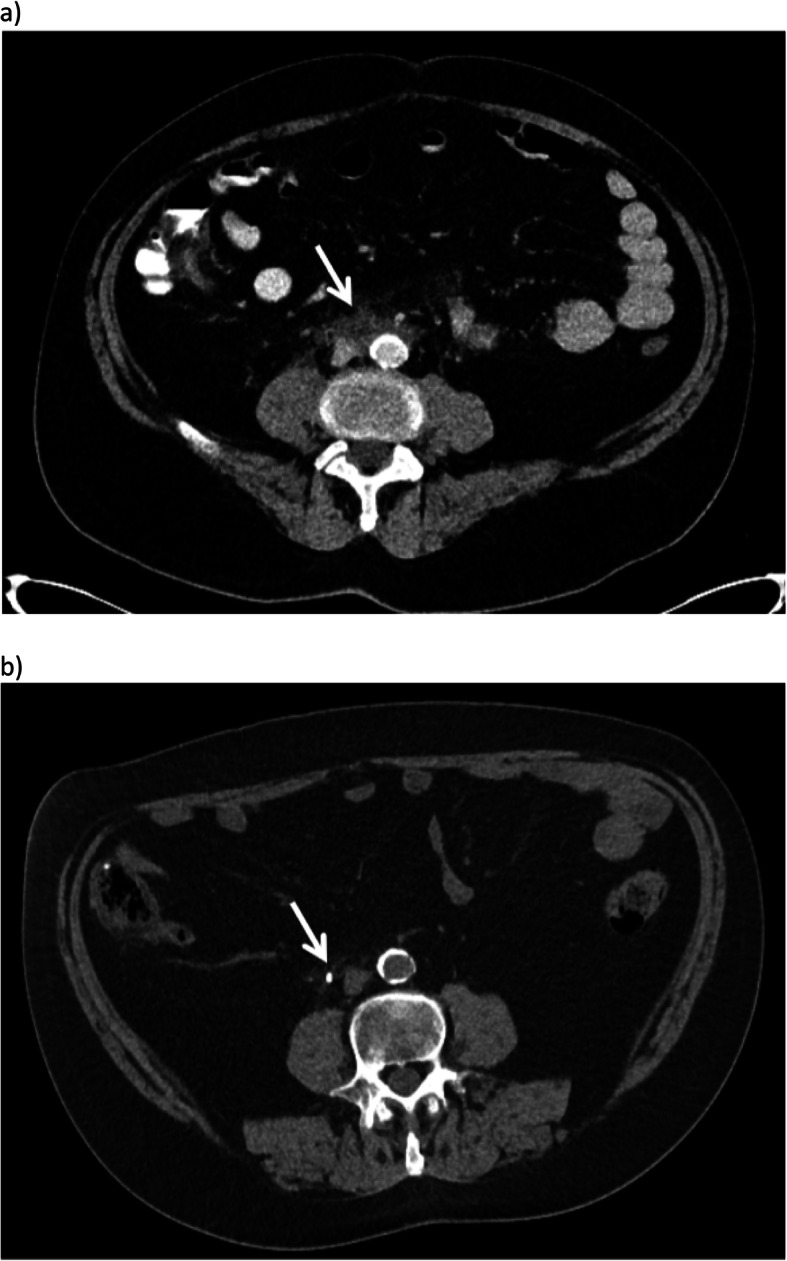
CT scan of Patient 2 pre-treatment (a) demonstrating a soft tissue mass (arrow) in keeping with RPF partially encasing the aorta, IVC and the right ureter. CT scan performed subsequently in the same patient post treatment (b) demonstrates that the soft tissue representing RPF has almost completely resolved. There is only a small amount of residual tissue (arrow) surrounding the right ureter, which has a stent in place
